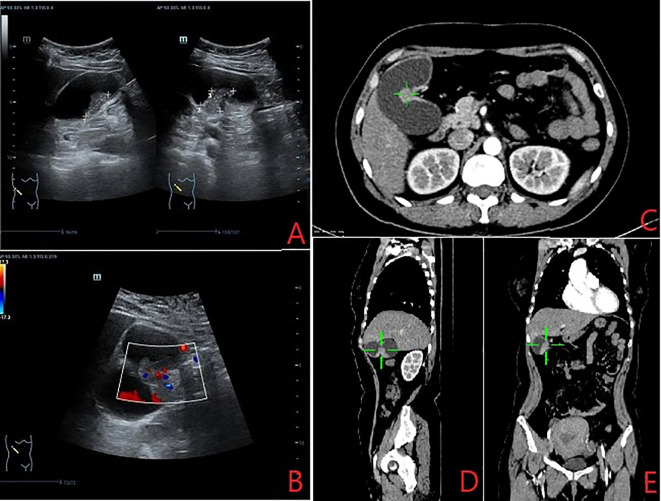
Preoperative imaging of gallbladder mixed adenoneuroendocrine carcinoma (GB-MANEC). (A, B) Abdominal ultrasonography. (A) Grayscale ultrasound revealed a heterogeneously isoechoic mass (calipers) occupying the gallbladder lumen, measuring approximately 3.0 cm × 2.5 cm × 2.0 cm, with an irregular contour. (B) Color Doppler imaging demonstrated prominent intralesional blood flow within the mass, indicative of hypervascularity. (C–E) Contrast-enhanced computed tomography (CT) viewed in three orthogonal planes. (C) The axial plane showed the tumor with irregular margins and heterogeneous enhancement (label “+”). (D) The sagittal plane further characterized the tumor’s morphology and its posterior extension (label “+”). (E) The coronal plane clearly delineated the extent of the mass (label “+”) and its relationship with the adjacent liver parenchyma.
